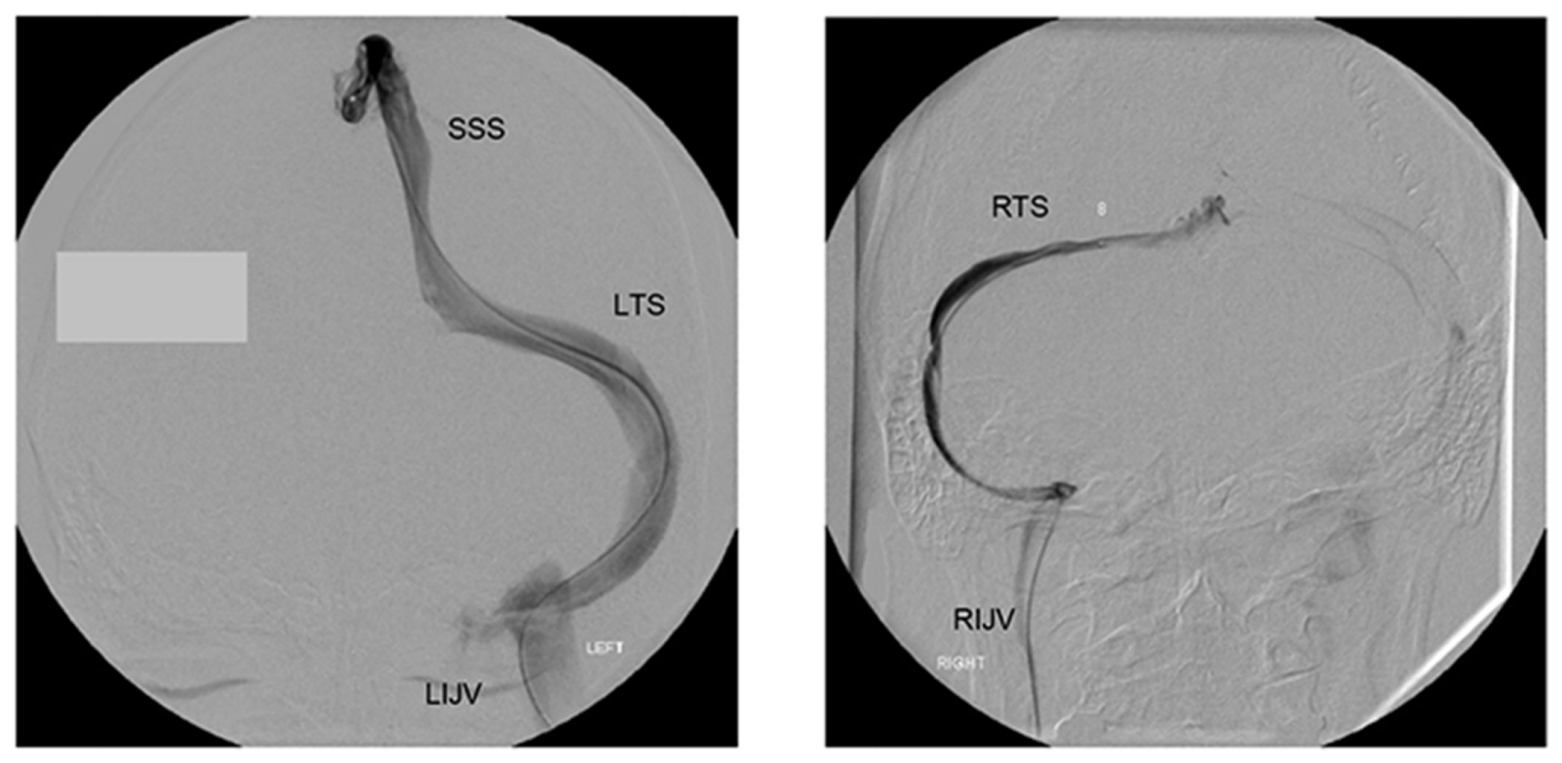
Catheter venogram, frontal view Left image shows radiographic contrast injected through a catheter in the sagittal sinus (SSS) draining exclusively into the left transverse sinus (LTS) and then the left internal jugular vein (LIJV). Right image shows a contrast injection from a catheter positioned in the right transverse sinus (RTS): the right transverse and sigmoid sinuses are small, as is the right internal jugular vein (RIJV).
